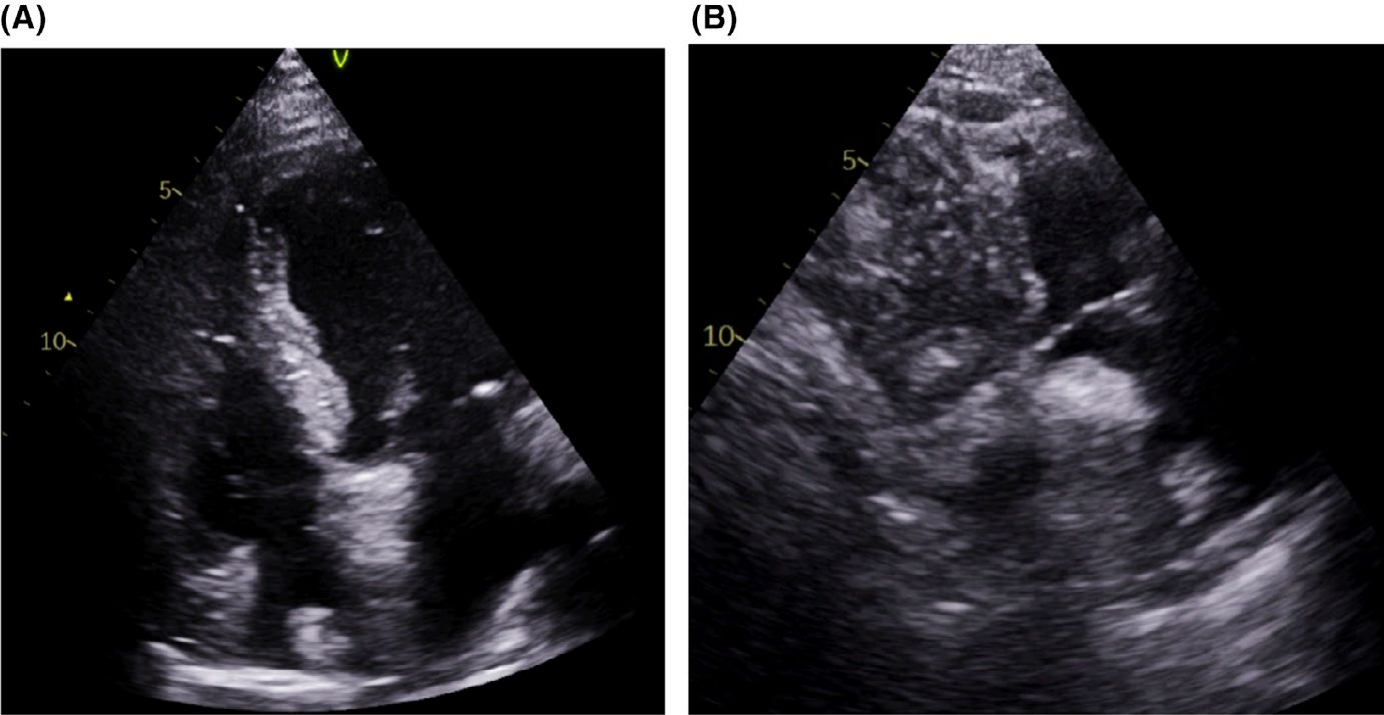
Transthoracic echocardiography (TTE). A, Modified apical four‐chamber view showing a right atrial mass (arrow). B, Right ventricle inflow view showing a large mass protruding into the tricuspid valve annulus (arrowhead)
